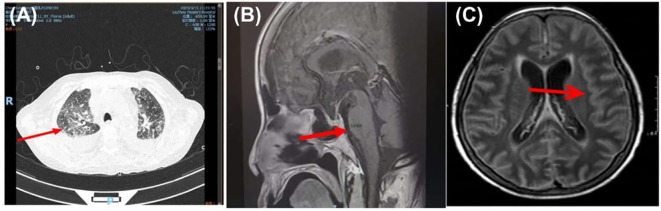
Radiological findings of the patient. (A) Chest CT showing bilateral pulmonary infiltrates (red arrow). (B) Sagittal pituitary MRI indicating a partially empty sella (red arrow). (C) Brain MRI demonstrating diffuse leptomeningeal enhancement (red arrow), consistent with infectious meningitis.
